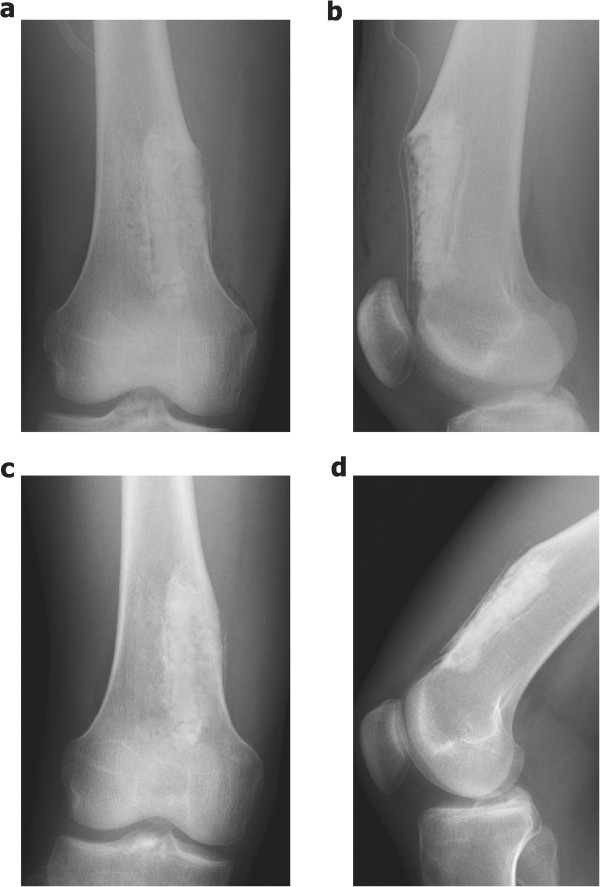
Postoperative plain radiographs. (a) Anteroposterior and (b) lateral postoperative routine radiographs showing the bone defect filled with IP-CHA and covered with a bioresorbable plate. (c) Anteroposterior and (d) lateral radiographs at 12-month follow-up showing no local recurrence of the tumor. Particles of IP-CHA were almost consolidated.
